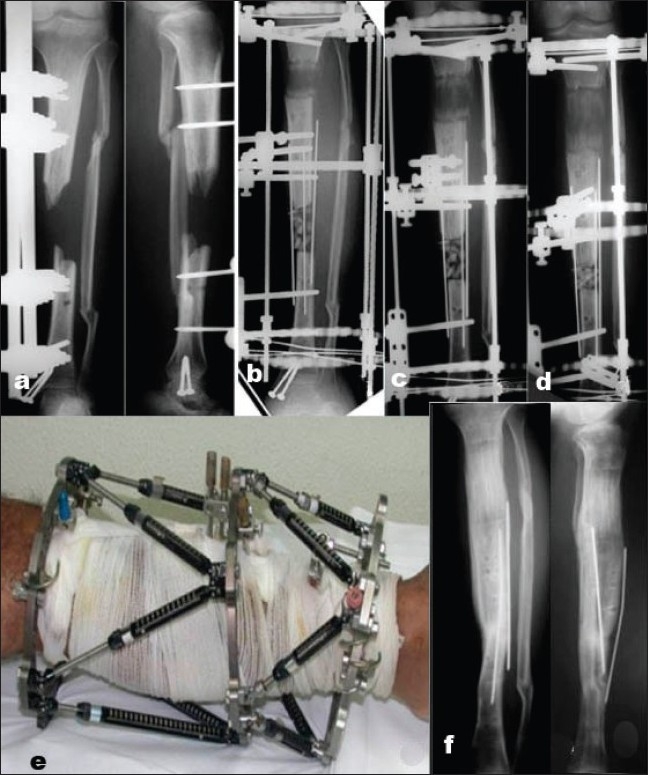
(a) Anteroposterior radiographs of open tibial shaft fracture with bone loss (case 14). (b,c,d) Anteroposterior radiographs after application of the TSF and progress of bone transport. (e) Clinical picture with TSF rings and Struts. (f) Anteroposterior and lateral radiographs 3 months after fixator removal with consolidated bone transport, healed docking site, and anatomic alignment is present.
